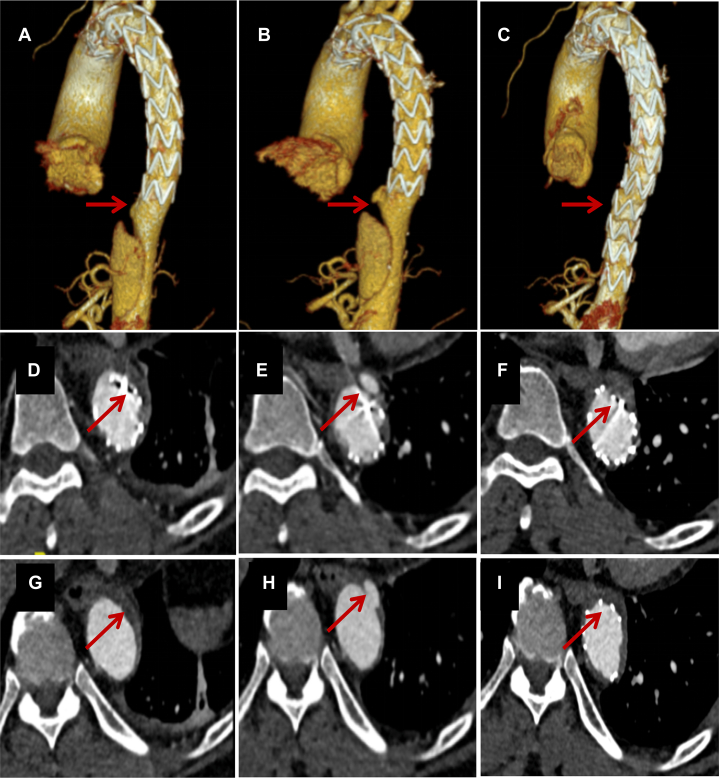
Longitudinal Computed Tomography Angiography Assessment of the Descending Aorta (A, D, G): Initial post-thoracic endovascular aortic repair imaging showing no distal dissection; (B, E, H): 1-month follow-up revealing a newly developed distal intimal tear below the prior stent graft, driving dissection progression.Red arrows show imaging changes before, after progression, and following surgery. (C, F, I): 3-month follow-up after the “one-stop” procedure, demonstrating complete resolution of the dissection and stable stent graft placement, validating the mechanical success of the secondary intervention.
